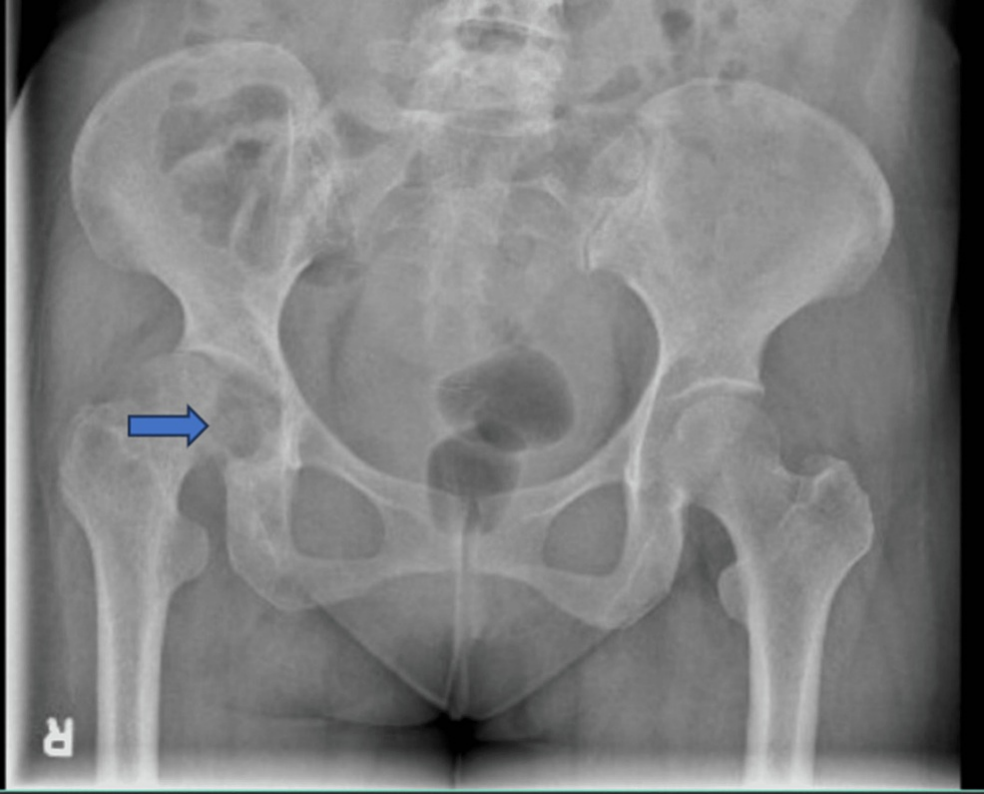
X-ray (anteroposterior view) of the pelvis with both hip joints showing lytic lesion in the right acetabulum (blue arrow).
